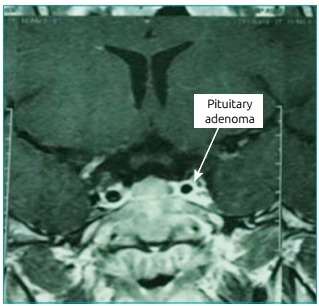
Magnetic Resonance Imaging of Turcica 3 TESLA: Dynamic study
